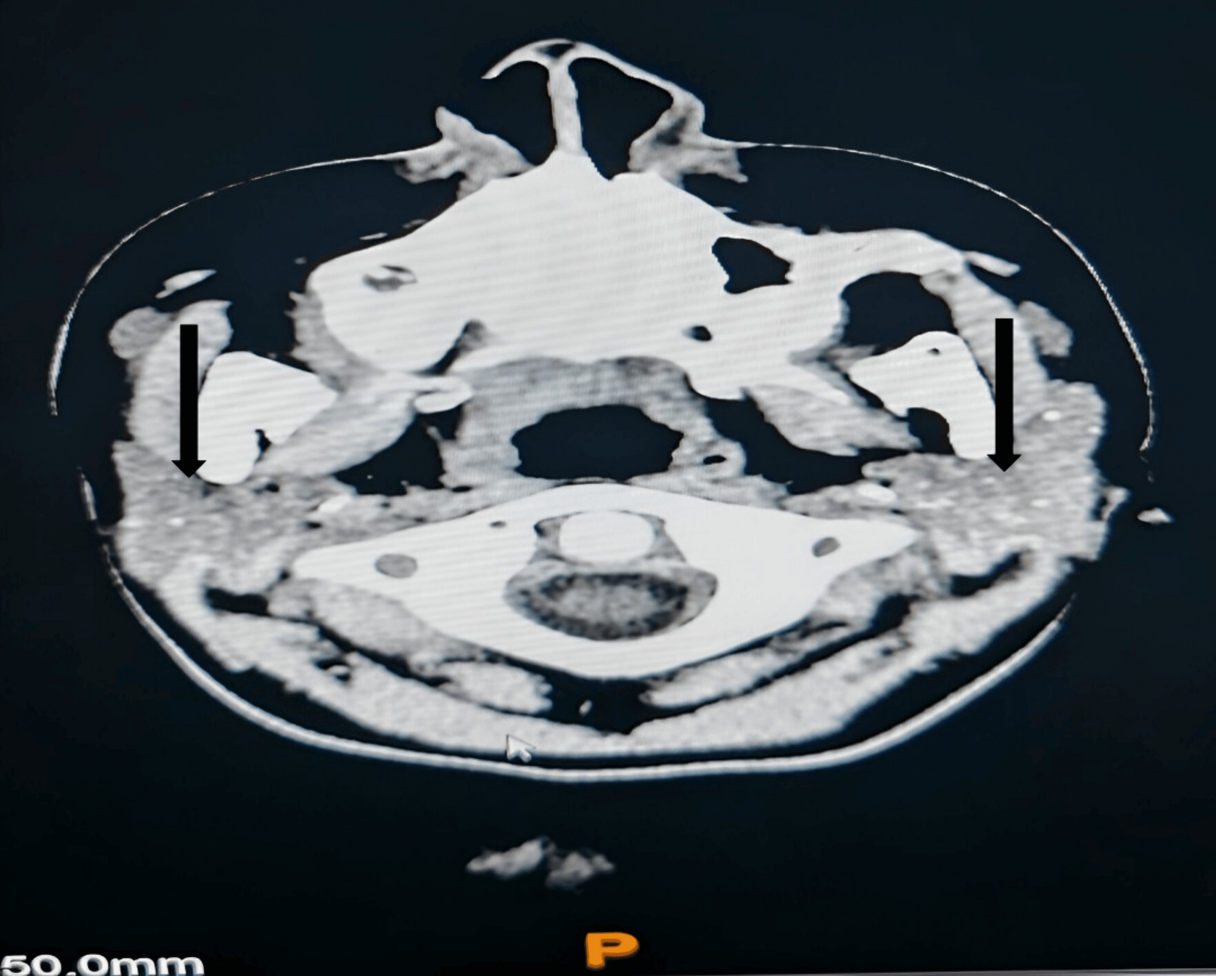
CT brain shows calcification within bilateral parotid gland (arrows).
